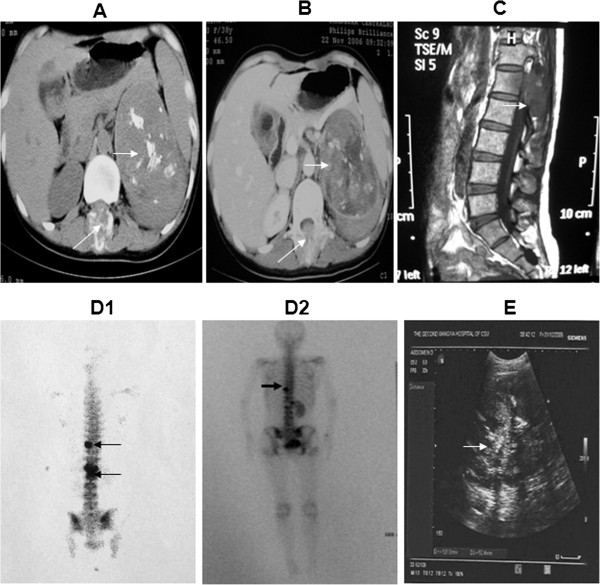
CT scan (A) and contrast enhanced CT scan (B) showed an inhomogeneous density of mass (10.5×6.7 cm^2^) in the left adrenal region, with cystic changes, scattered calcified foci (arrow) and inhomogeneous enhancement (arrow). The mass is ventral to the left kidney and the abdominal aorta. (C) MRI showed a well-defined mass and showed a mass in the back of vertebra (arrow). (D1) SPECT showed Tc-99m-MDP highly accumulated in the tenth of thoracic vertebra and the first and second lumbar (arrow). (D2) SPECT showed that the whole body bone scintigraphy is clear, with no abnormal structure detected. On the left half of the ninth thoracic a radionuclide uptake dot is visible. Left renal agenesis is seen (arrow). (E) Ultrasonographic study further demonstrates the mass has an uneven internal echo (arrow). The mass is irregular in shape and without a distinct boundary, between the left kidney and spleen.
